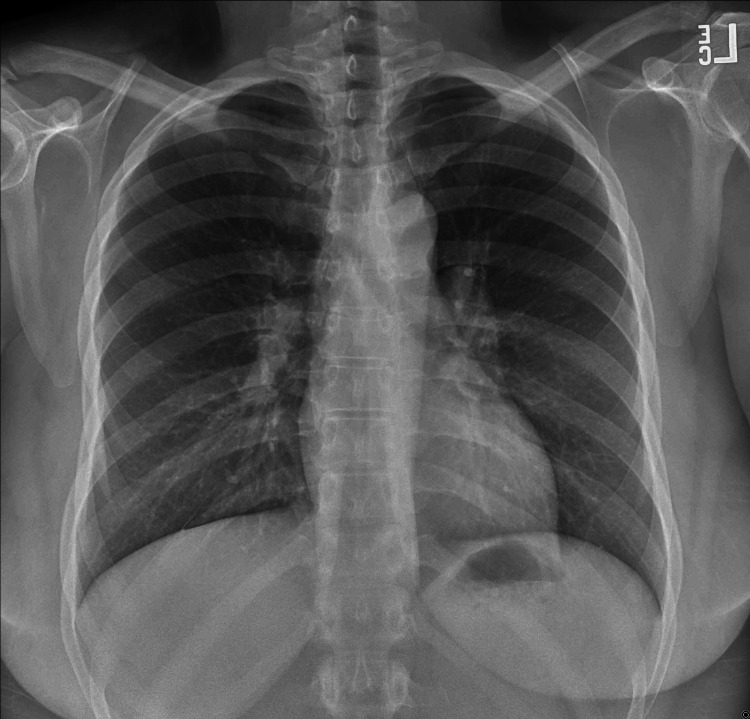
Anteroposterior (AP) chest X-ray
